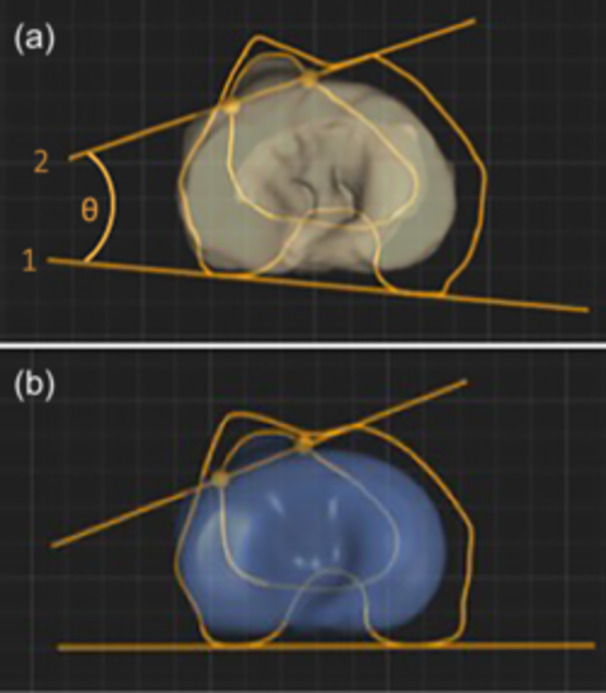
Calculation of the tibial tubercle (TT) torsion angle. (a) A patient image is used to manually calculate TT torsion using an axial computed tomography (CT) slice. (1) The cut in which the posterior femoral condyles are best visualized is used to create the posterior femoral condylar axis. (2) To determine the TT axis, a line is drawn through the intersection of two points marking the medial and lateral borders of the tibial tubercle. (θ) The TT torsion angle is then calculated on the medial side of the two axes. (b) Example of TT torsion calculation using three‐dimensional bone modelling of CT scans from SOMA automated software (24.2°).
